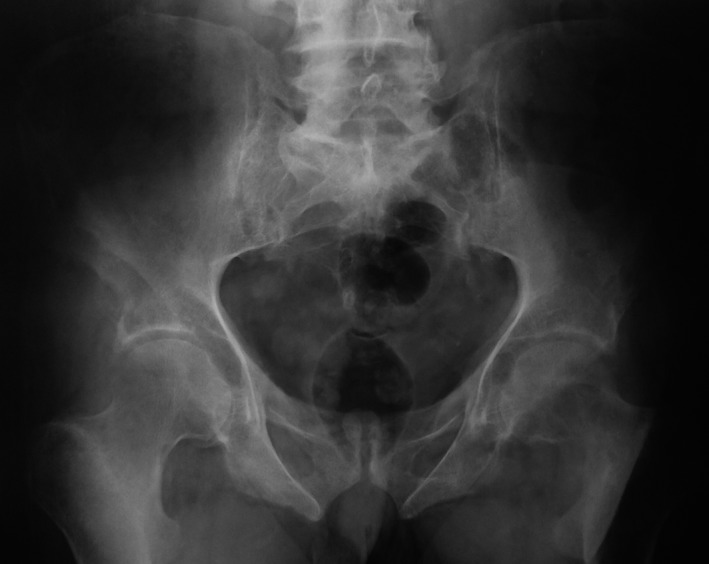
Radiograph of the pelvis: lytic lesion of the left femoral neck.
